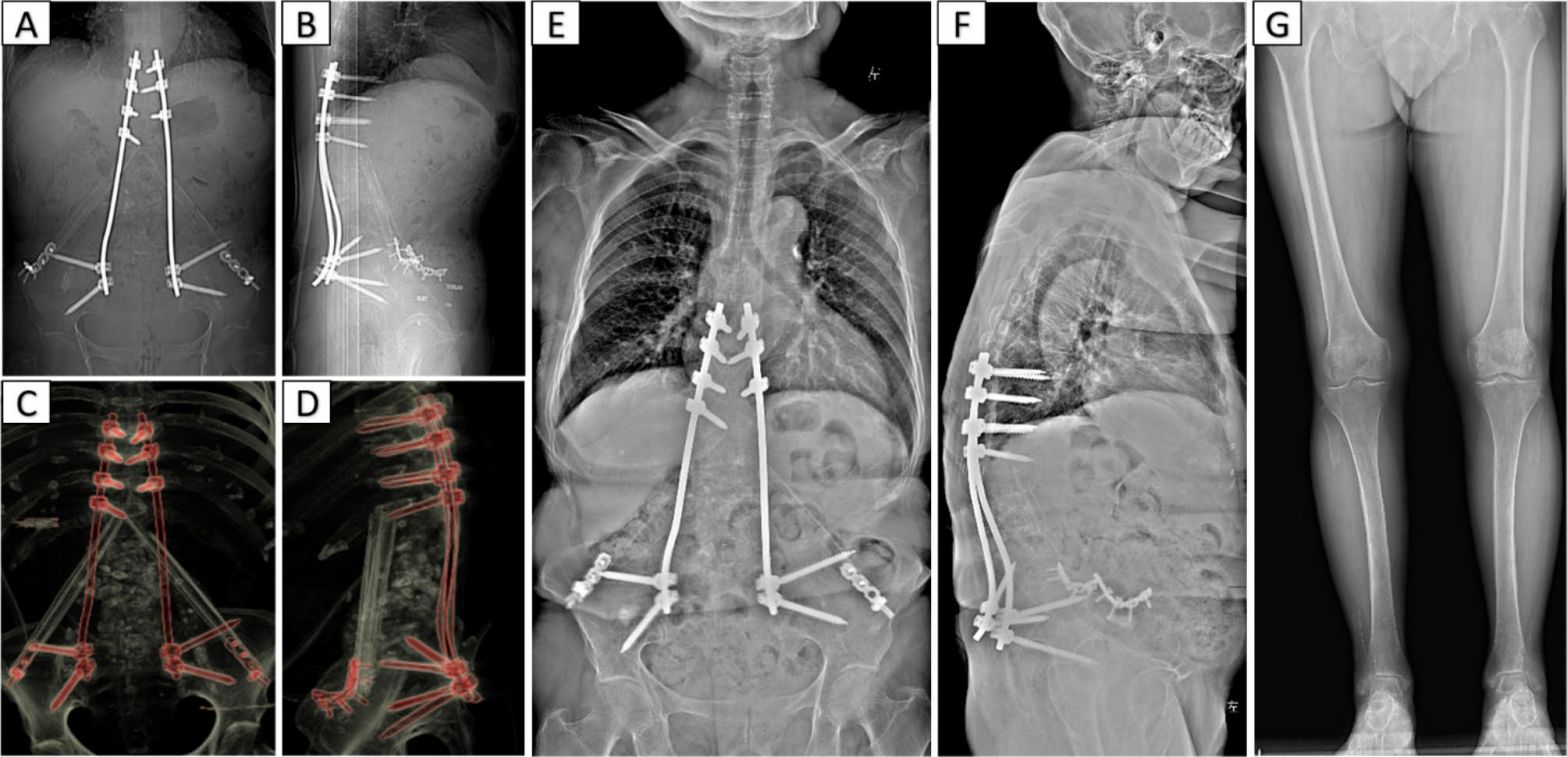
(A and B) Postoperative X-ray examination. (C and D) Postoperative CT examination. (E and F) The most recent postoperative X-ray examination of the full length of the spine. (G) X-ray examination of the full length of the lower limb after surgery showed partial absence of the fibula
